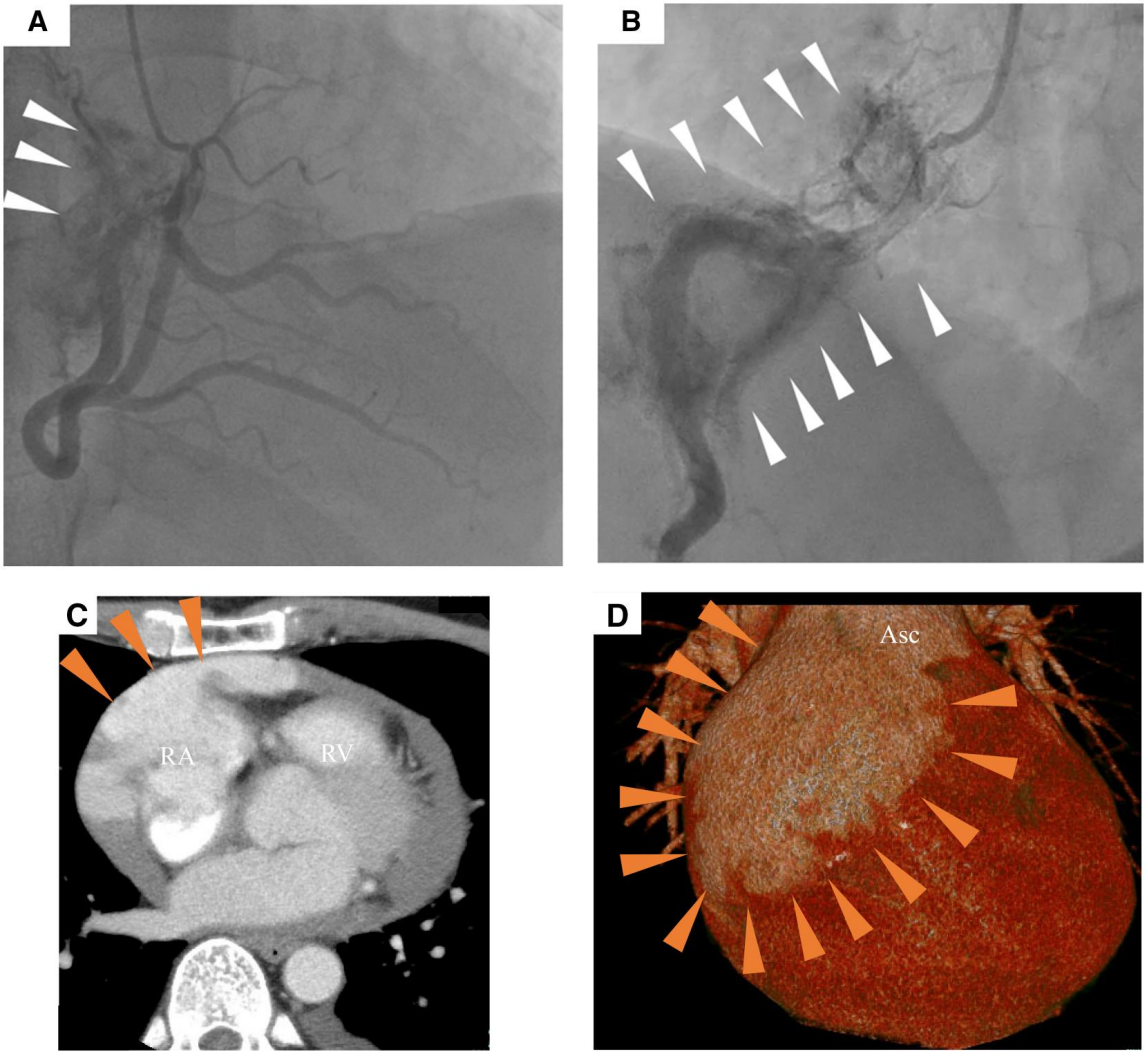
(A) Coronary angiography (CAG: left anterior oblique) presenting collateral vessels from the right coronary artery (RCA) to the right atrium (RA) (white arrows). (B) During the third cardiac tamponade, the CAG displays further RA enlargement with structural collapse, without RCA stenosis (white arrows). (C) Contrast-enhanced computed tomography reveals an enlarged RA and RA rupture-induced massive pericardial effusion due to RA rupture (red arrows). (D) Three-dimensional computed tomography reveals an RA rupture-induced hematoma and RA tissue, extending to the front of the ascending aorta (red arrows) RA, right atrium; RV, right ventricle; Asc Ao, Ascending Aorta
